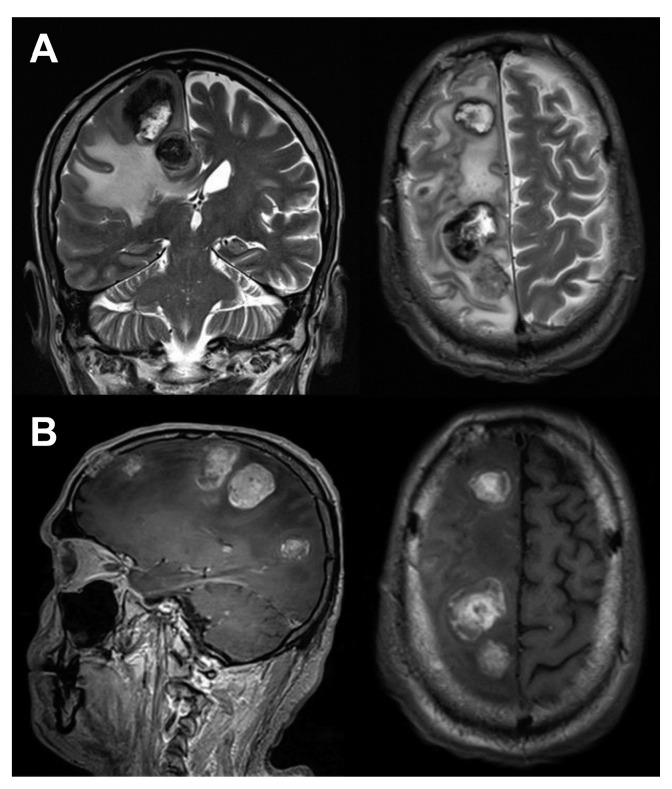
MRI of the brain performed subsequently to 18F-FDG-PET/CT. (A) Coronal and transaxial T2 images; (B) Sagittal and transaxial T1 TSE images. The study showed multiple metastatic lesions, widespread throughout the brain, hypointense on T2 and hyperintense on T1 images.Moreover, a large edema in the white matter of the right cerebral hemisphere and a lesion in the frontal bone infiltrating the meninx were observed. Therefore, due to extensive brain metastases [9,10,11,12] and worsening of treatment adverse effects [4], immunotherapy was discontinued and the patient was subjected to the best supportive care until his conditions progressively worsened to death after a few months.
